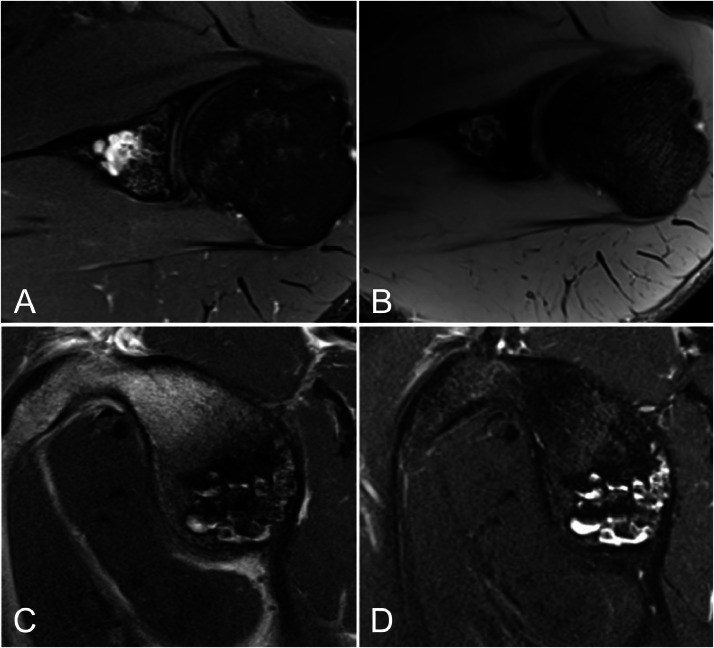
MRI of the left shoulder with (A) proton density fat-saturated axial, (B) T2-weighted gradient echo axial, (C) proton density sagittal, and (D) T2-weighted fat-saturated sagittal sequences. Multiple gas-fluid levels are demonstrated. There is no evidence of intra-lesional fat.
